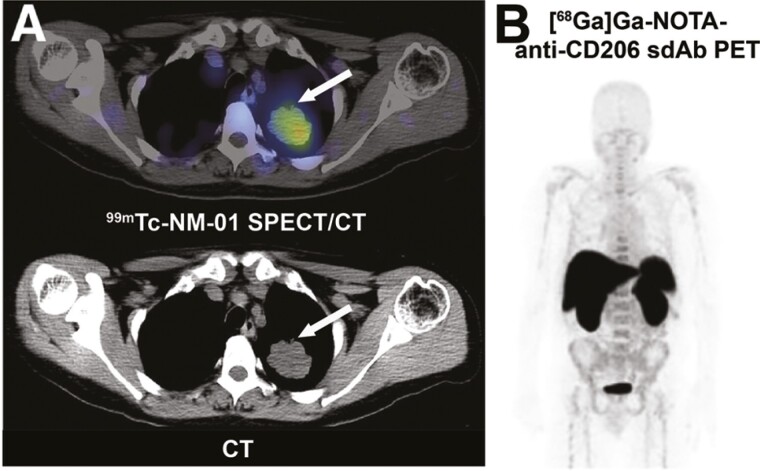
Examples of the clinical applicability of nanobody-based molecular imaging of programmed death ligand 1 and the macrophage mannose receptor (CD206). (A) Axial SPECT/CT (top) and corresponding CT-imaging (bottom) of the programmed death ligand 1 (PD-L1) targeting and ^99m^Tc-labeled nanobody NM-01 in a patient with a high PD-L1-expressing primary non-small cell lung cancer (arrow). (B) Maximum-intensity projection [^68^Ga]Ga-NOTA-anti-CD206 Nb (MMR3.49) images of a non-small cell lung cancer patient at 1.5 h post-injection. CT: computed tomography; ^68^Ga: gallium-68; PET: positron emission tomography; SPECT: single-photon emission computerized tomography; ^99m^Tc: technetium-99m. Images have previously been published in an adapted form by: (A) *JNM.* Xing *et al*. Early Phase I Study of a ^99m^Tc-Labeled Anti-Programmed Death Ligand-1 (PD-L1) Single-Domain Antibody in SPECT/CT Assessment of PD-L1 Expression in Non-Small Cell Lung Cancer. J Nucl Med. 2019;60(9):1213-1220. © SNMMI [65]; and (B) *JNM*. Gondry *et al*. Phase I Study of [^68^Ga]Ga-Anti-CD206-sdAb for PET/CT Assessment of Protumorigenic Macrophage Presence in Solid Tumors (MMR Phase I). J Nucl Med. 2023;64(9):1378-1384. © SNMMI [22], under a CC BY 4.0 license.
